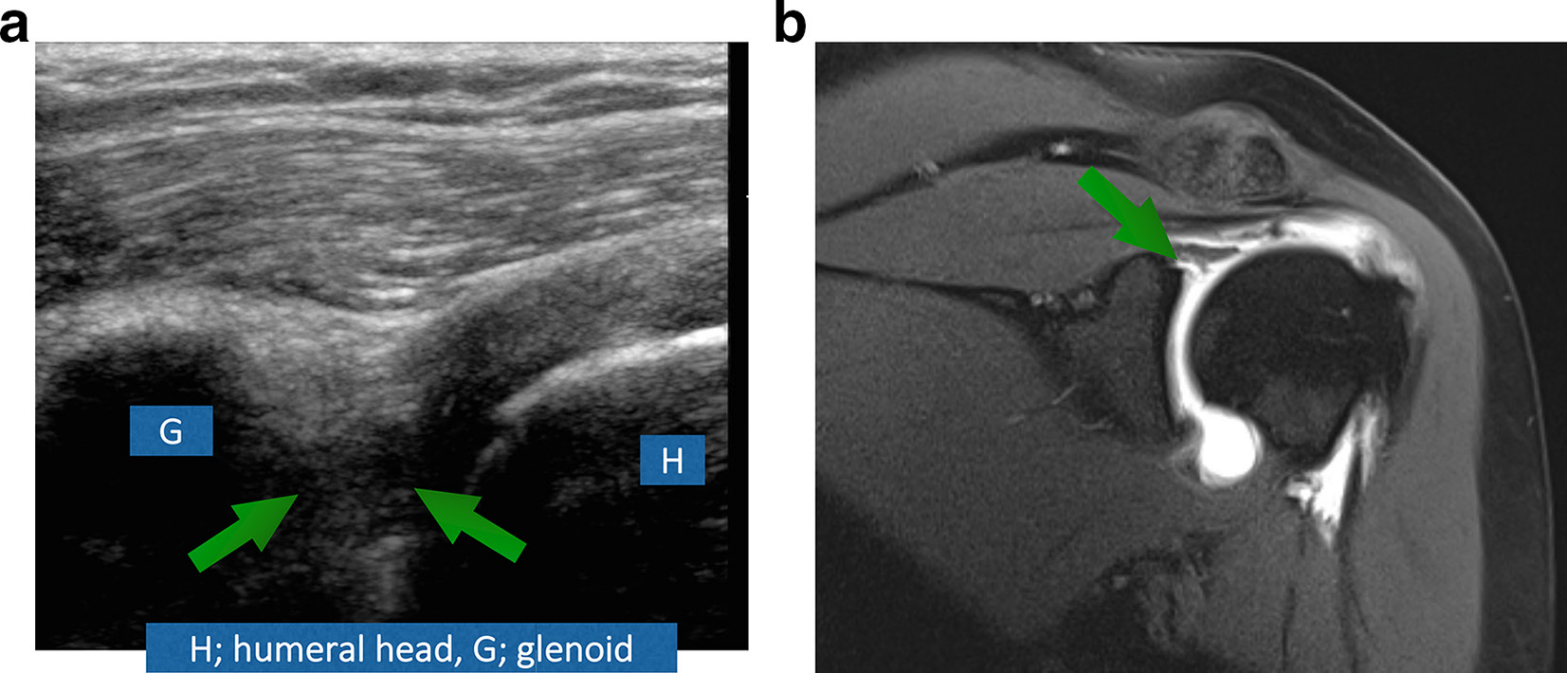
(A) A 37-year-old male patient with history of trauma. Ultrasound (Figure 5A) shows hypoechoic tear (Greenarrow) extending in the labrum parallel to its attachment to the bony glenoid in keeping with SLAP Type 2 lesion. Same findings are seen on MRI (Figure 5B).
